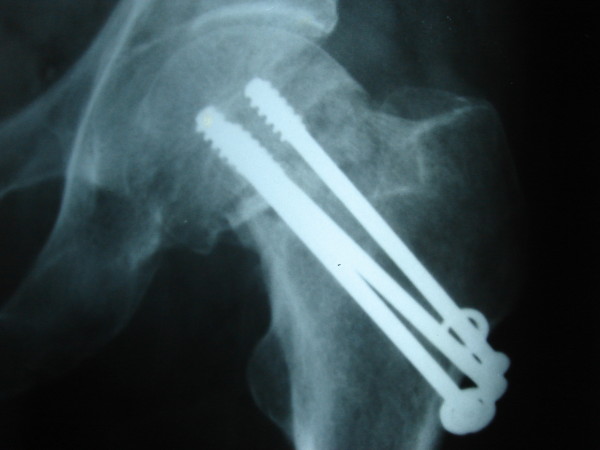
Case no 2, radiograph at 6 months, showing displacement.
